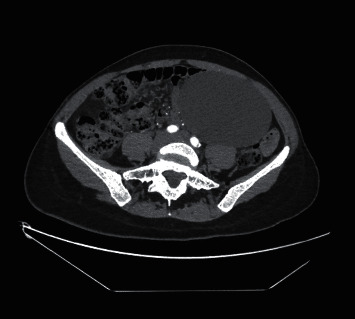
CT scan with a cystic derivative in the left lower abdominal quadrant.
